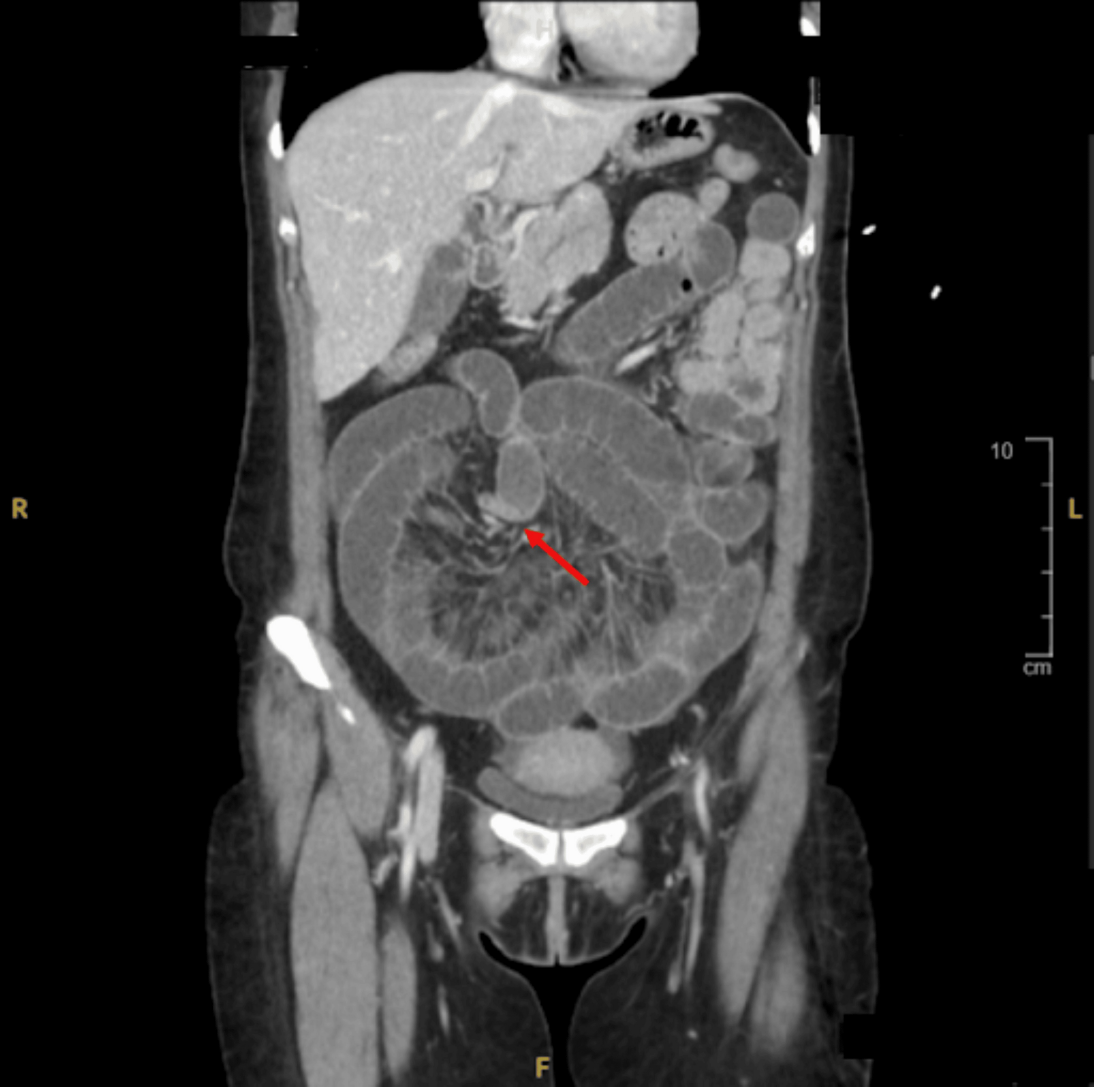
Coronal CT image of the abdomen demonstrating a transition point (red arrow) at an angulated loop of small bowel in the mid to upper abdomen, consistent with SBO. Proximal bowel loops are fluid-filled and dilated, whereas distal loops appear decompressed. SBO, small bowel obstruction
